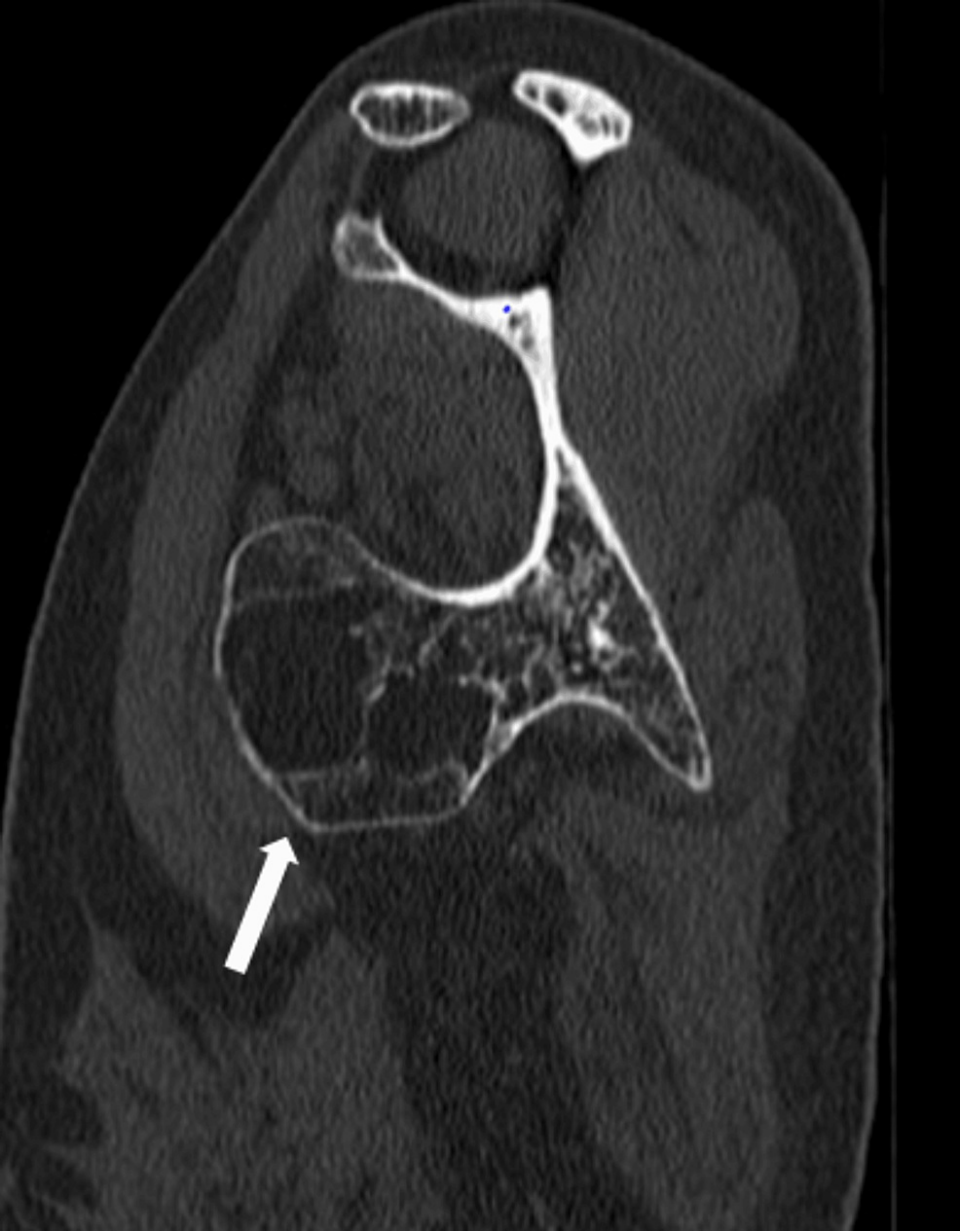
CT scan showing the bony mass with the stalk attached to the scapula CT: computed tomography
